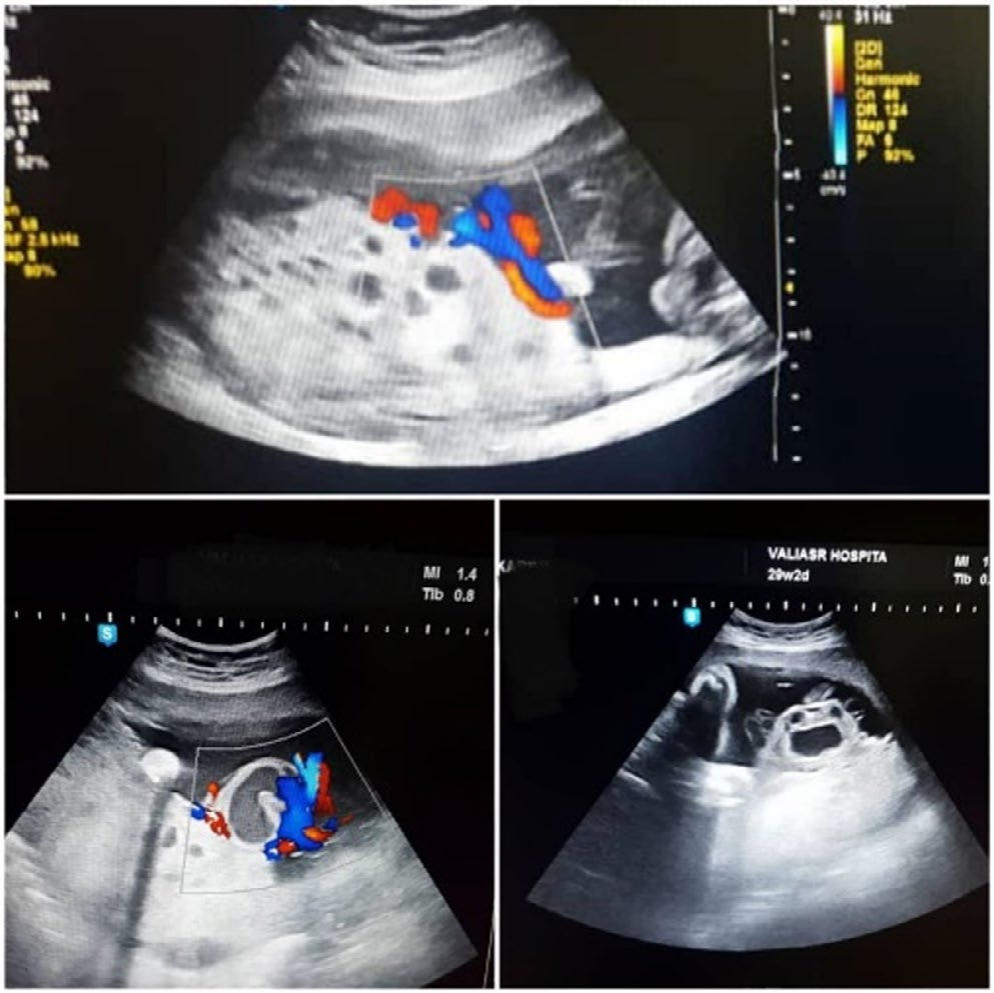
Upper image shows cystic appearance of the placenta and lower image shows the varix of umbilical vein in color Doppler (right side) and Gray scale Imaging (left side)
